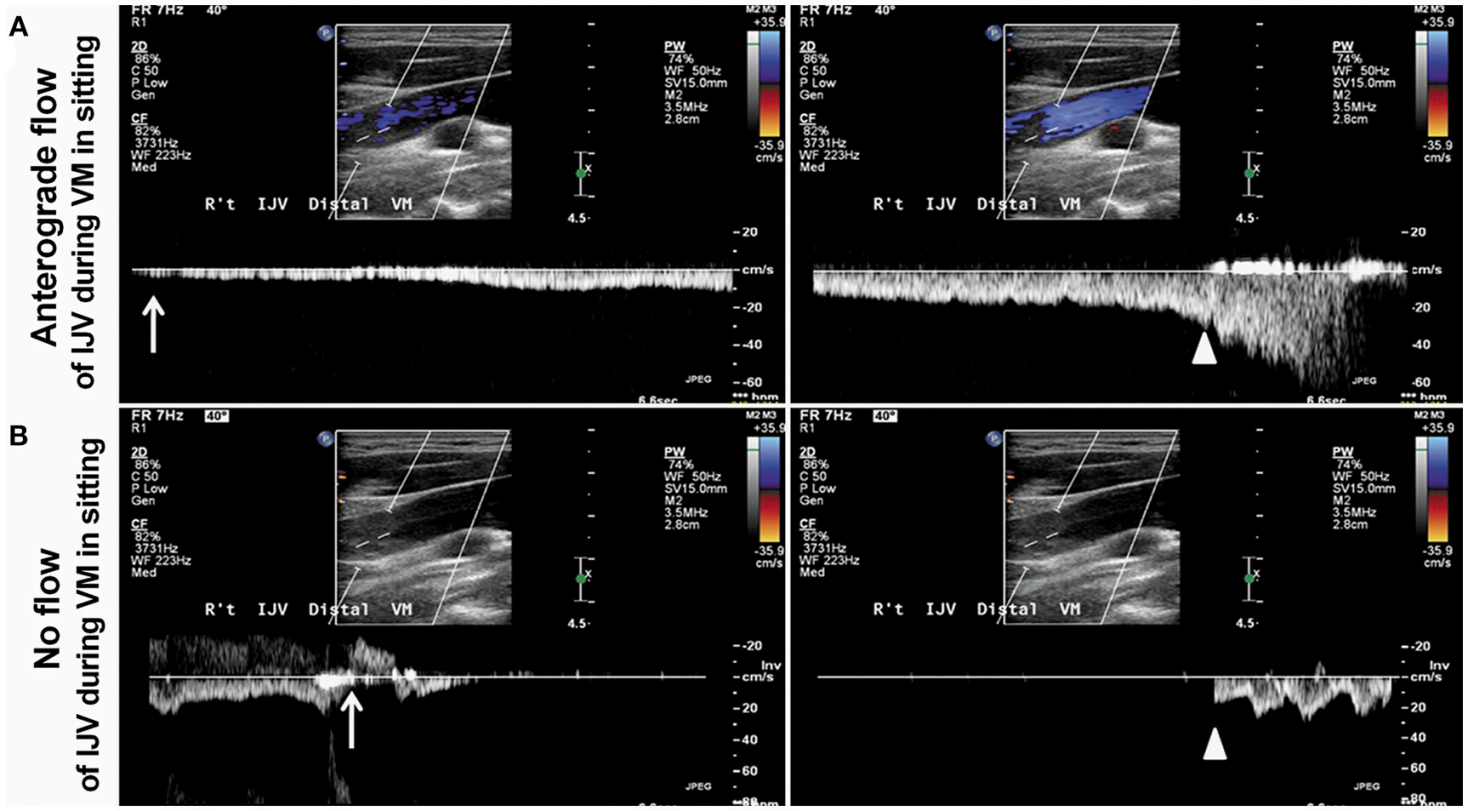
Display of Doppler spectra of the IJV (internal jugular vein) during the VM (Valsalva maneuver) in the sitting position. (A) Patient 1. An anterograde flow in the right IJV during the VM in the sitting position (upper A) suggests that the pressure can be released by reopening the IJV in a 42-year-old healthy female with patent IJV. (B) Patient 2. No flow in the right IJV during the VM in the sitting position (lower B) with a transient venous reflux (arrow, B) indicates that the intracranial venous pressure cannot be relieved effectively by the reopening the IJV in a 53-year-old TGA patient with significant IJV stenosis/obstruction. The arrows indicate the beginning of the VM, and the arrowheads indicate the end of the VM. The figure was reproduced with the permission of Han et al. (16).
